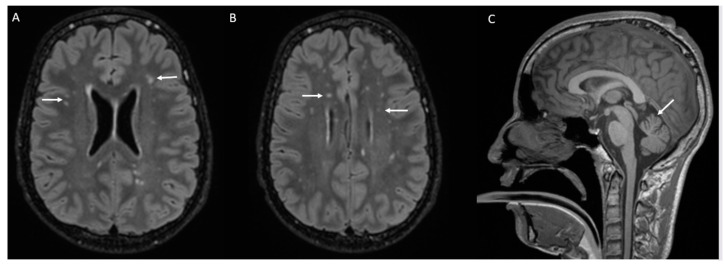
(A,B): The axial FLAIR-weighted image shows multiple small hyperintensity signals in the deep white matter, with a radial distribution around the lateral ventricles, predominantly in the bilateral fronto-parietal regions (arrow). (C): The sagittal T1-weighted image shows mild atrophy of the superior cerebellar vermis (arrow).
